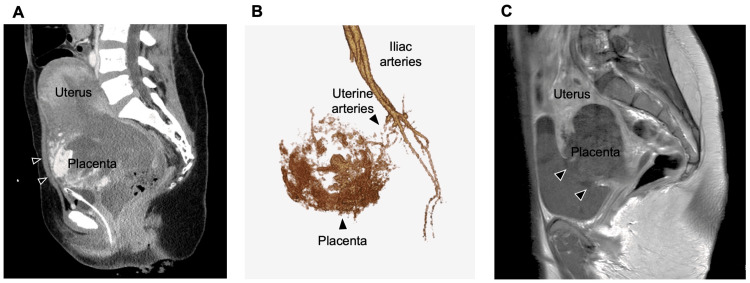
Imaging evaluation of the residual placenta and penetration into the bladder A. Enhanced CT image shows active extravasation from the residual placenta (arrow). B. 3D-CT angiography image of the hypervasculated placenta fed by the uterine artery. C. MRI of perforation of the placenta into the bladder (arrow) and clot retention in the bladder
